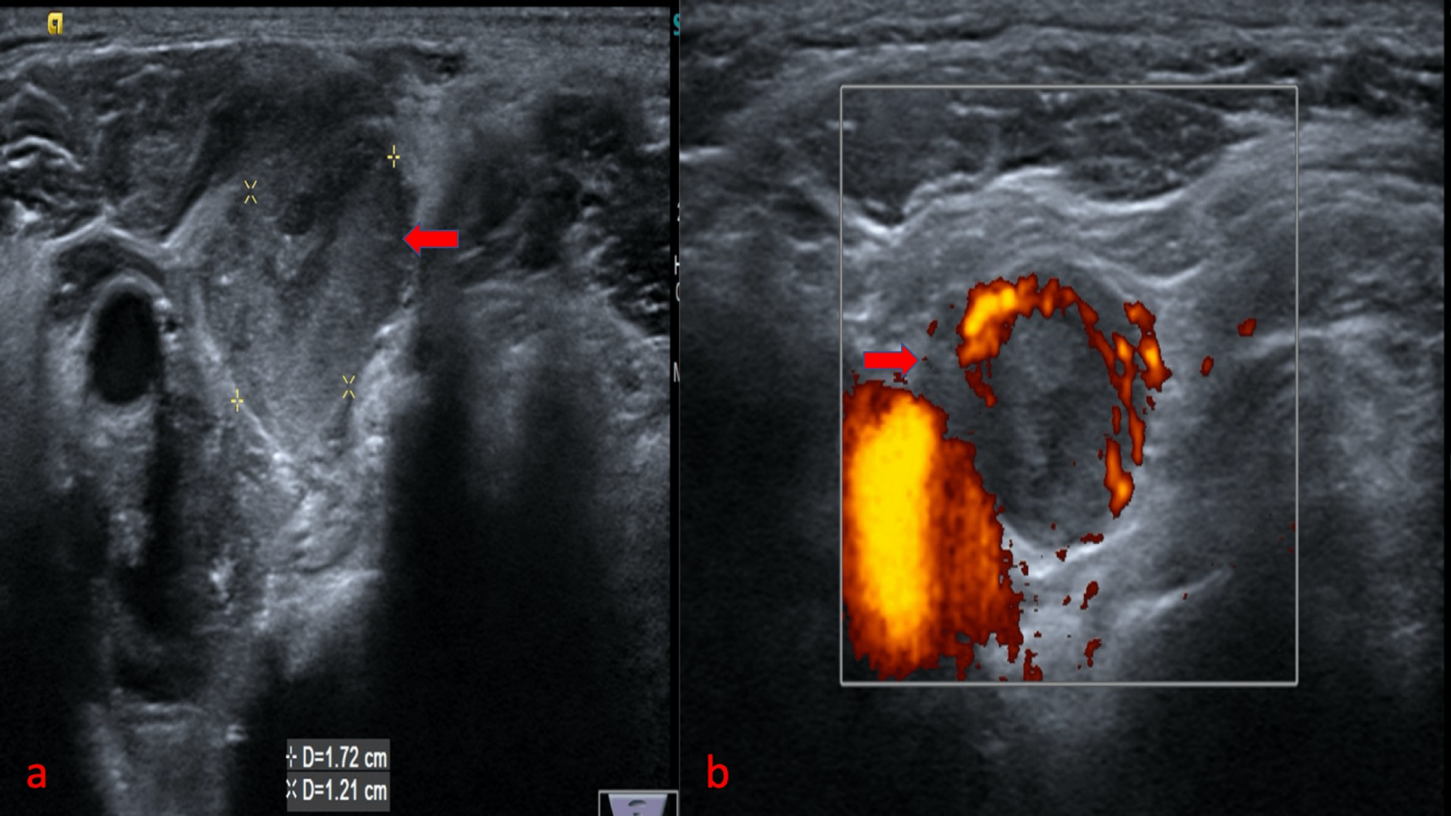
Imaging of metastatic cervical lymph node from Carcinoma hypopharynx: (a) BMUS shows an enlarged heterogeneously hypoechoic node with loss of fatty hilum (red arrow), (b) CDUS shows peripheral vascularity in the node (red arrow) BMUS: B-mode ultrasound; CDUS: color doppler ultrasound
